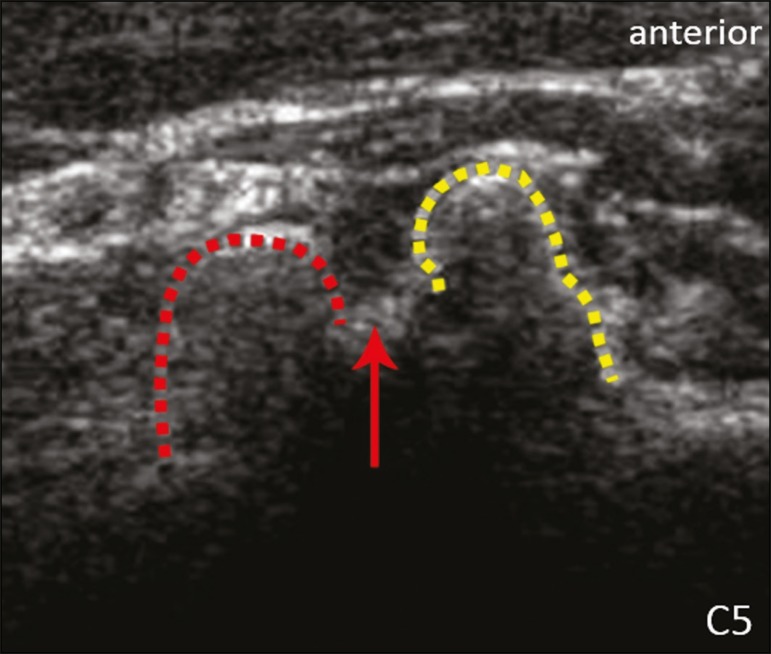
Ultrasound in the transverse plane, showing the C5 root (arrow), anterior
